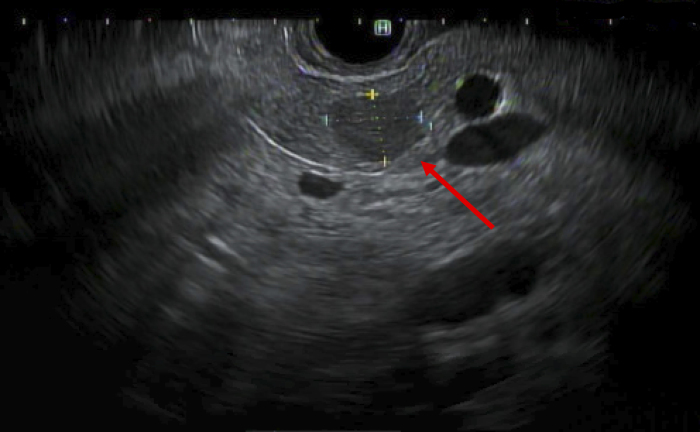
Endoscopic ultrasound demonstrating a well-circumscribed hypoechoic pancreatic lesion without pancreatic ductal dilation. Red arrow denotes the pancreatic lesion.
